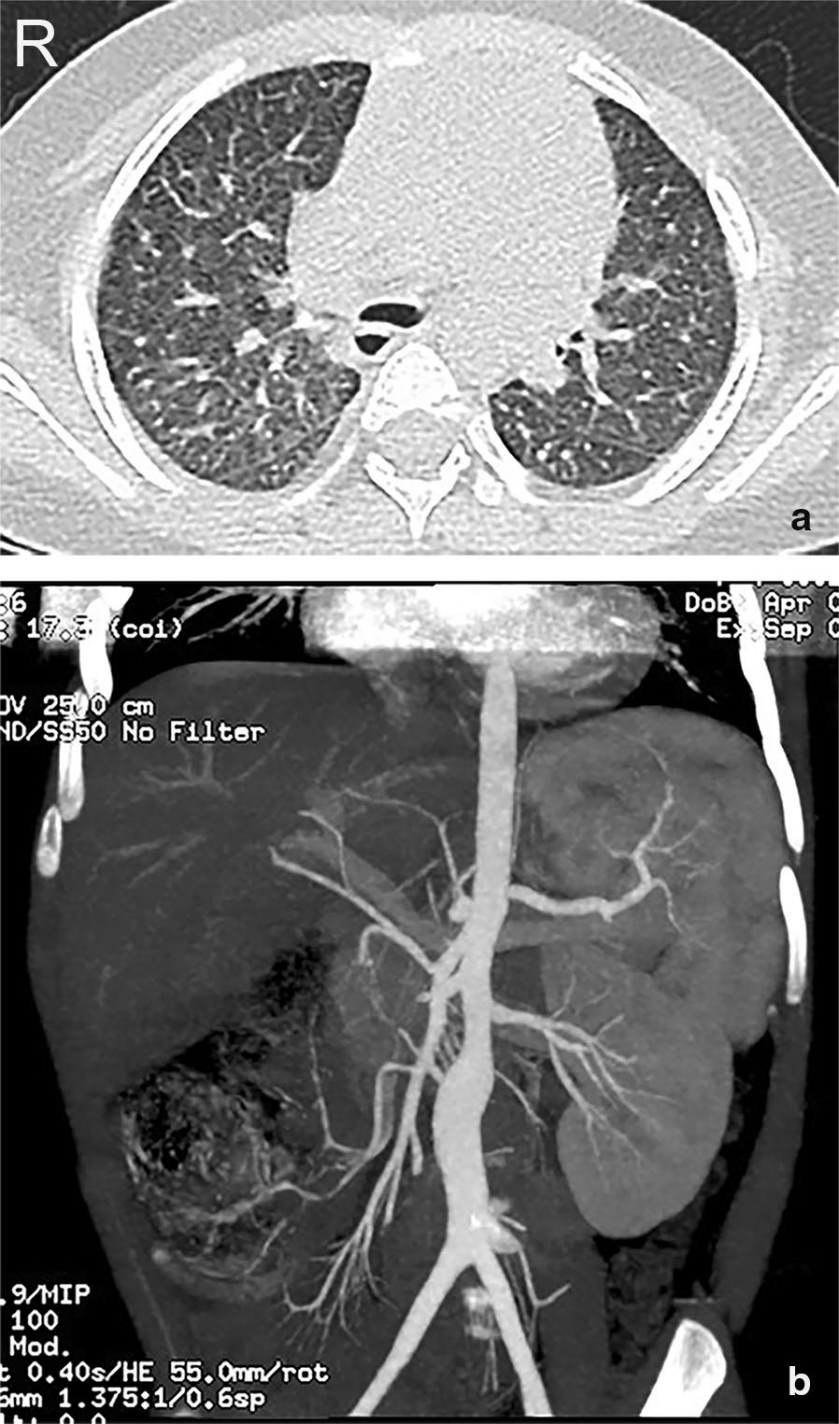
Chest and abdominal CT scan findings. a Extensively parenchymal and interstitial lesions of bilateral lungs are shown. b Thickened aortic wall, and stenosis and dilation of segmental abdominal aorta are shown
